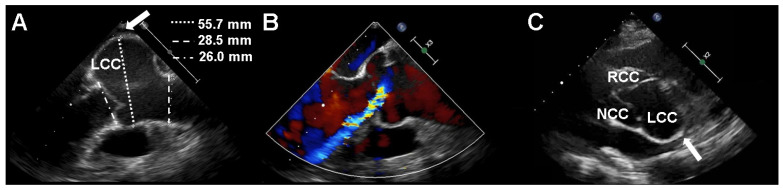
Transesophageal echocardiography (TEE) and transthoracic echocardiography (TTE) in the proband with Marfan syndrome. TEE (A) and (B) and TTE (C) revealed an isolated sinus of Valsalva aneurysm of the left coronary cusp (arrow) with severe aortic regurgitation. LCC, left coronary cusp; RCC, right coronary cusp; NCC, noncoronary cusp.
